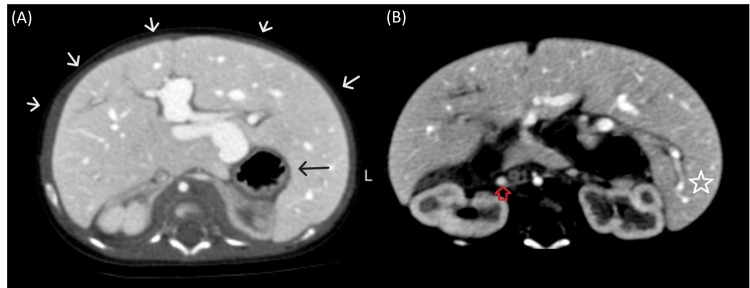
(A-B) Selected axial CT scan cuts at the level of the upper abdomen reveal a midline liver (white star and arrows), asplenia, left stomach (black arrow), and interrupted IVC (red arrow). CT: Computed tomography; IVC: Inferior vena cava
